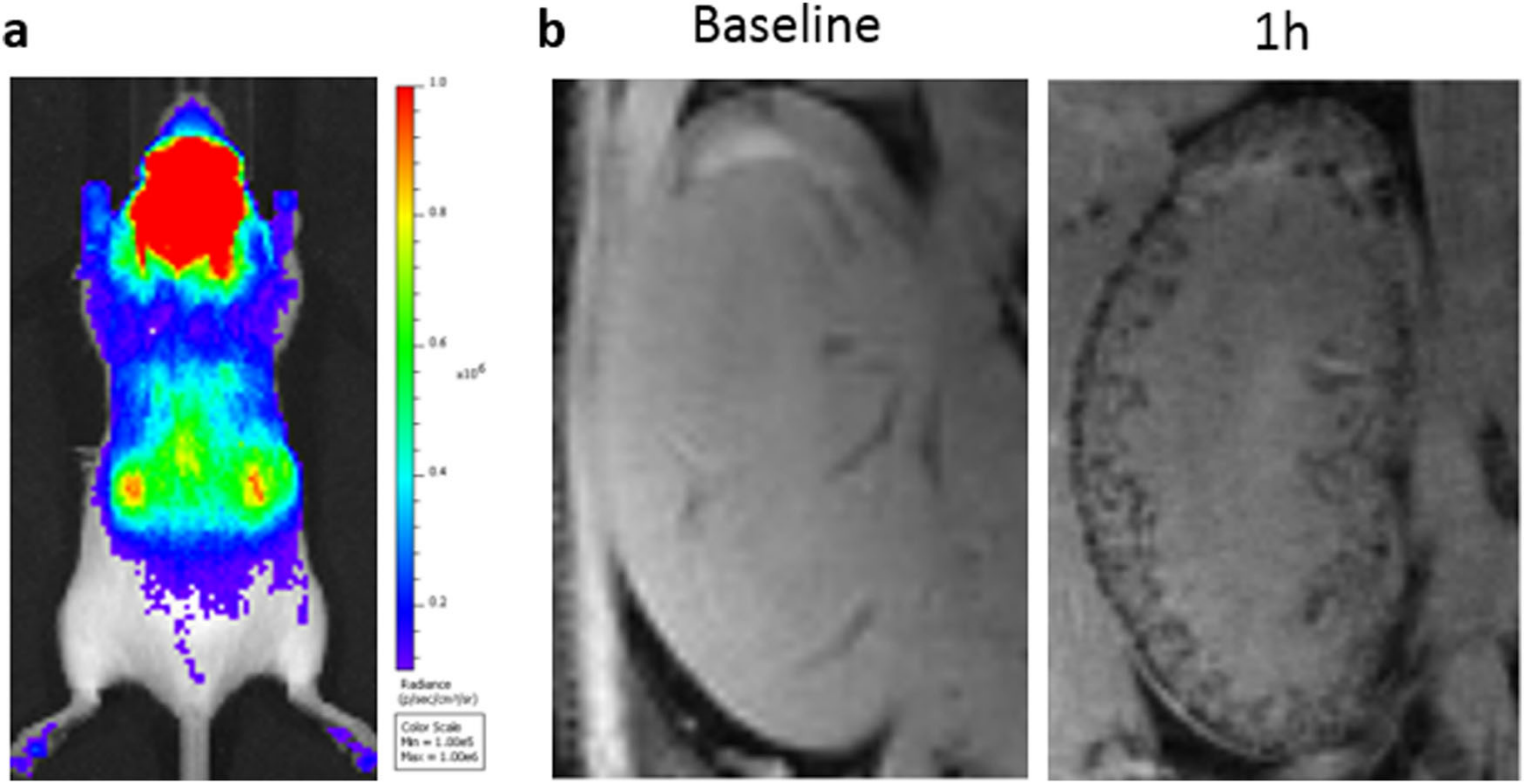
Multi-modal imaging of Luciferase^+^/SPION^+^ stem cells administered to the left cardiac ventricle. a BLI gives a fast confirmation of successful IC injection, and gives an approximate location of cells, but lacks organ-specific information. b MR imaging of the kidneys before and after the administration of SPION-labelled stem cells reveals that SPION-labelled cells are within the cortex of the kidney. Data generated at the Centre for Preclinical Imaging, University of Liverpool
